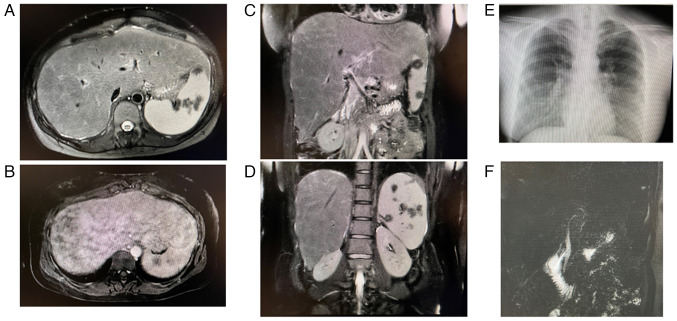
Pre-treatment MRI and posteroanterior chest X-ray. (A-D) MRI revealed extensive hepatosplenomegaly with multiple non-enhancing splenic nodules. (A, C and D) T2-weighted fat saturation-MRI; (B) gadolinium-ethoxybenzyl-diethylene-triaminepentaacetic acid-MRI late-stage. (E) A chest X-ray displayed no abnormal shadows in the lung field. (F) Magnetic resonance cholangiopancreatography demonstrated no stenosis or obstruction of the intrahepatic or extrahepatic bile ducts. MRI, magnetic resonance imaging.
